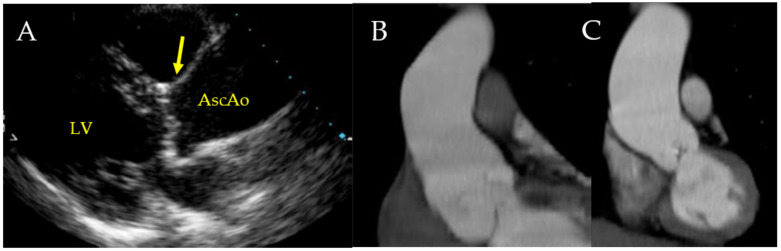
(A–C). Depicts representative findings on echocardiography and computed tomography (CT). In (A), the transthoracic echocardiogram shows normal dimensions of the sinuses of Valsalva (arrow) and a dilated ascending aorta. Ascending aorta denotes proximal ascending aorta, and LV denotes left ventricle. In (B,C), the CT images reveal dilatation of the aortic root and dilatation of the ascending aorta and proximal arch, respectively. Licenses Centre Cardiologique du Nord; order date 27 July 2022; order number 5357160571198; publication NEJM; Title: Aortic Dilatation in Patients with Bicuspid Aortic Valve.
